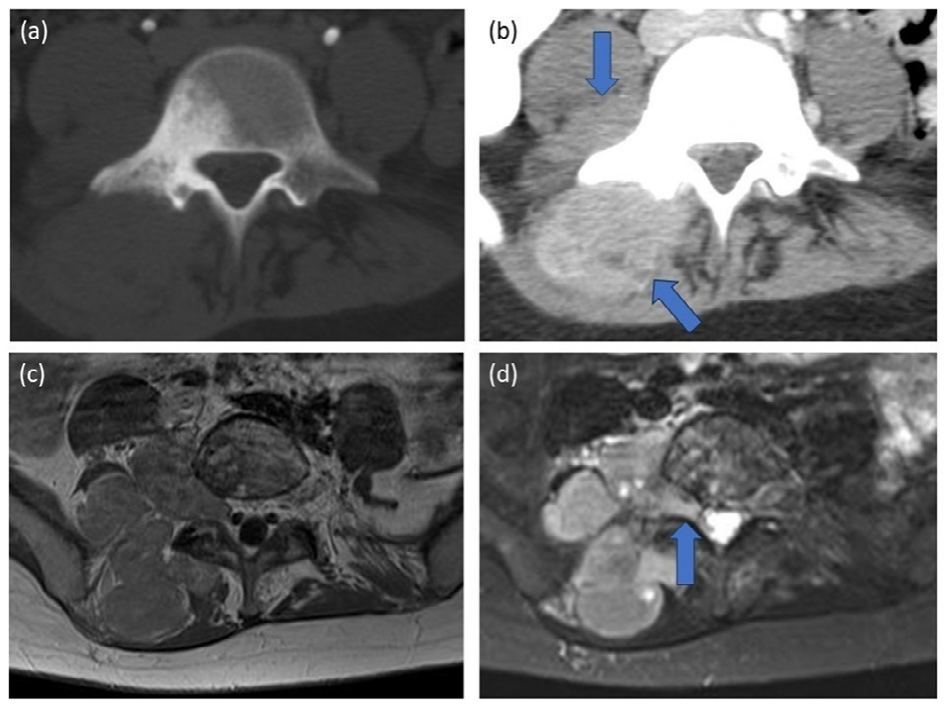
Primary diagnosis. (a, b) Abdominal CT. An irregular sclerotic lesion in hemicorpus L5, with extensive associated paravertebral soft tissue involvement (5.5 × 6.5 × 6.0 cm) (arrows). (c, d) Spinal MRI. A T2-hyperintense, heterogeneously contrast-enhancing mass in hemicorpus L5, with associated soft tissue involvement extending into the intervertebral foramen L4-L5 (arrow).
